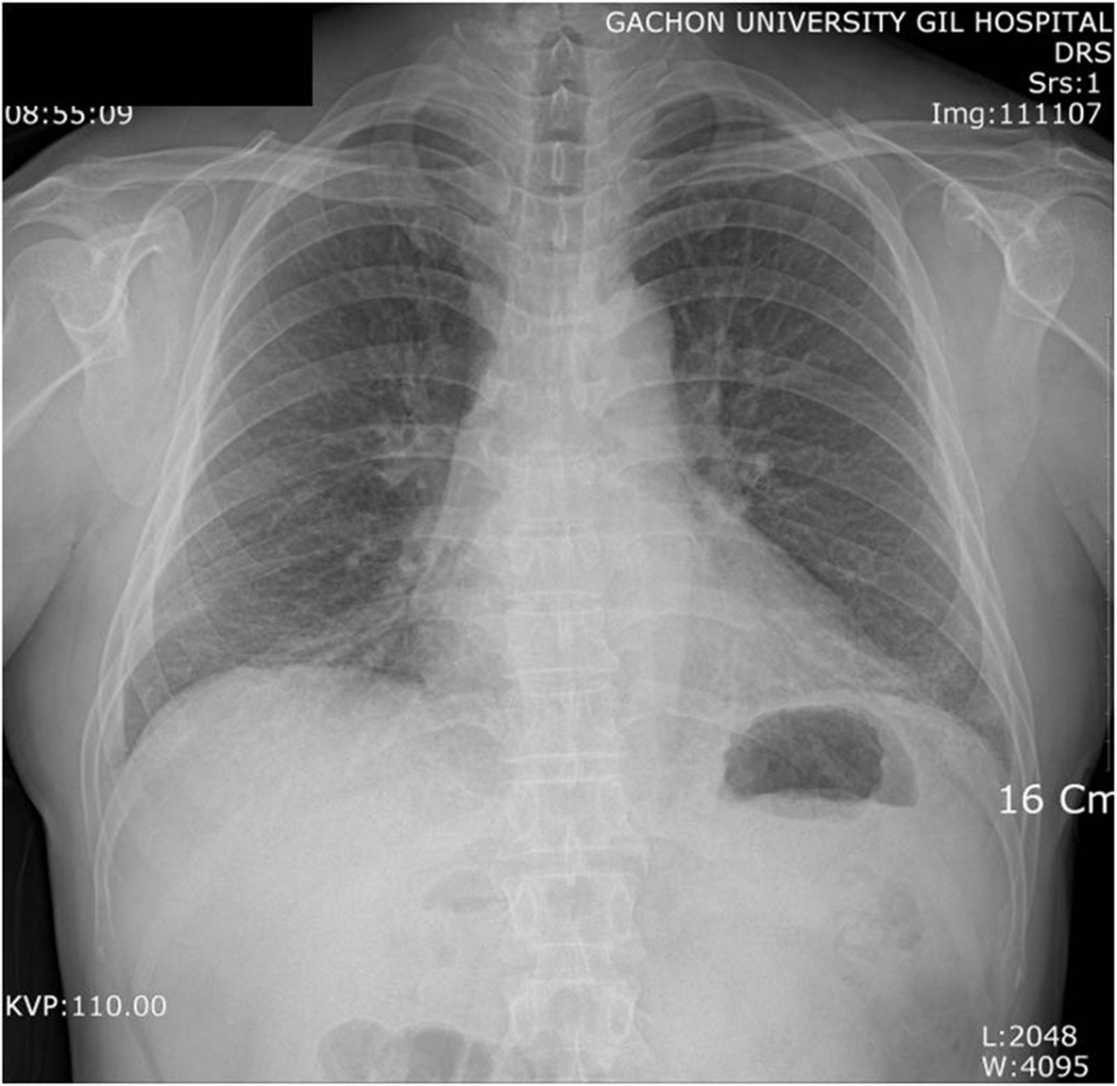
A chest radiograph. Ground glass opacities are seen in both lower lung fields
